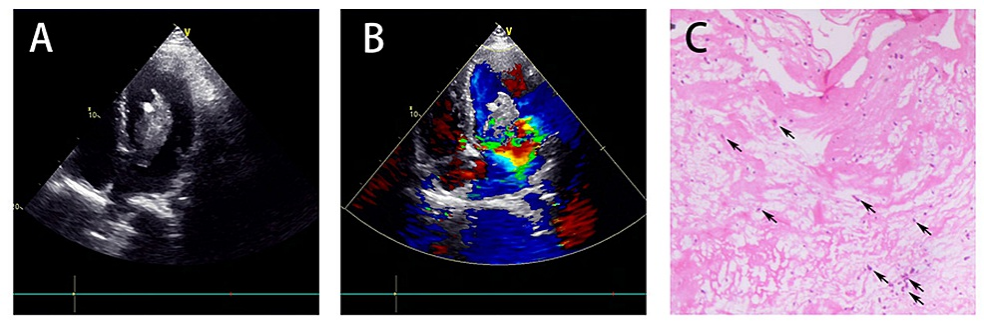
Echocardiographic and pathological findings (A and B) Echocardiography showed left atrial mass measuring 5.09 * 3.34 cm and moderate-to-severe mitral regurgitation. (C) Pathological examination of the mass tissue revealed stellate and spindle tumor cells (black arrow) surrounded by eosinophilic myxoid stroma.
